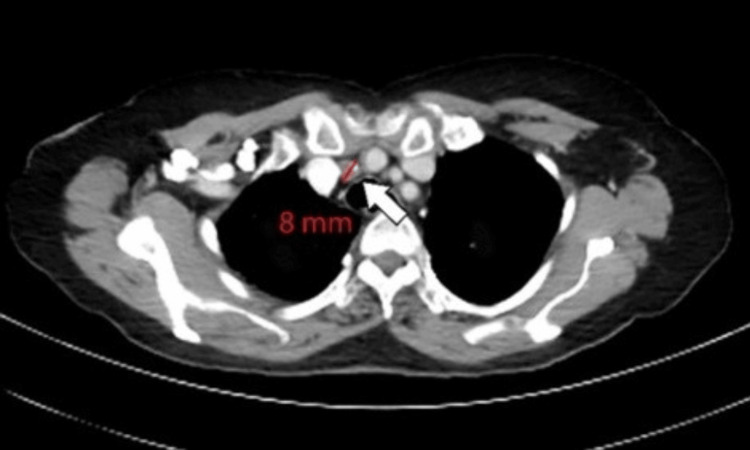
CT component showing a prominent pretracheal lymph node measuring 8 mm in dimension (arrow) CT: computed tomography
